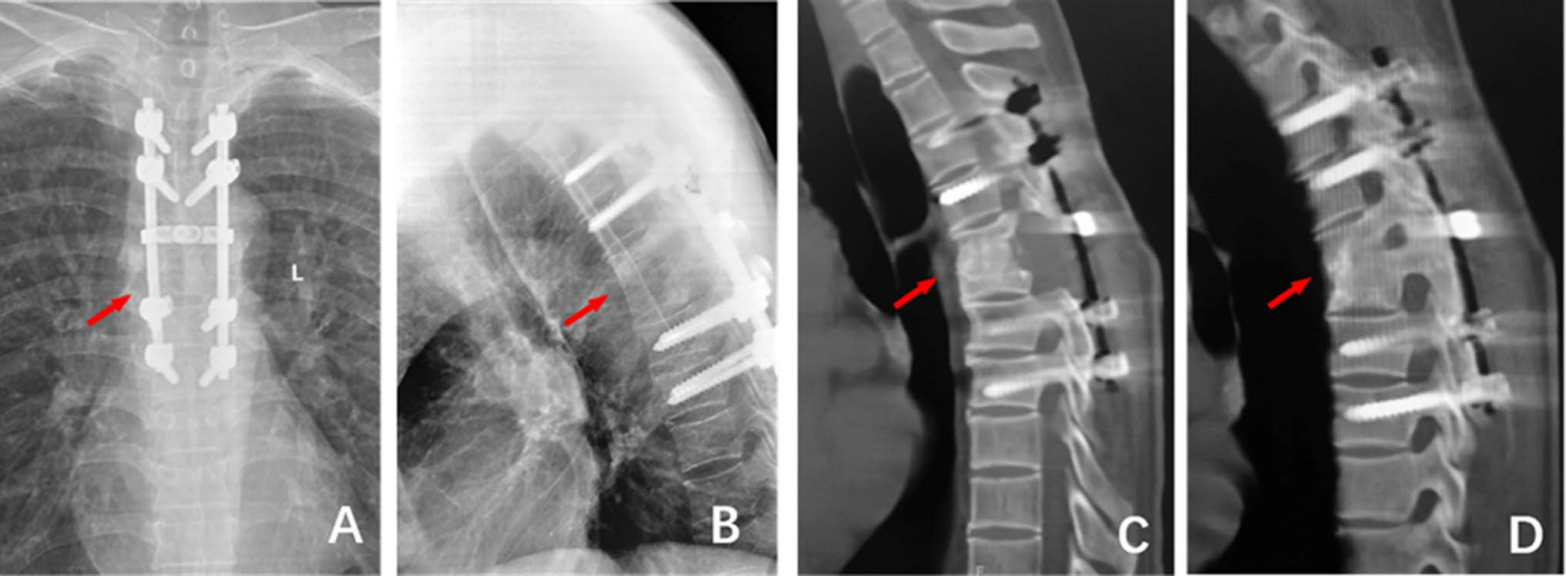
5 years after the operation, (A)and (B) The frontal and lateral X-ray films showed good internal fixation position, bone graft fusion, and Cobb Angle of 20°; (C) and (D): CT examination showed good internal fixation position and bone graft fusion.
